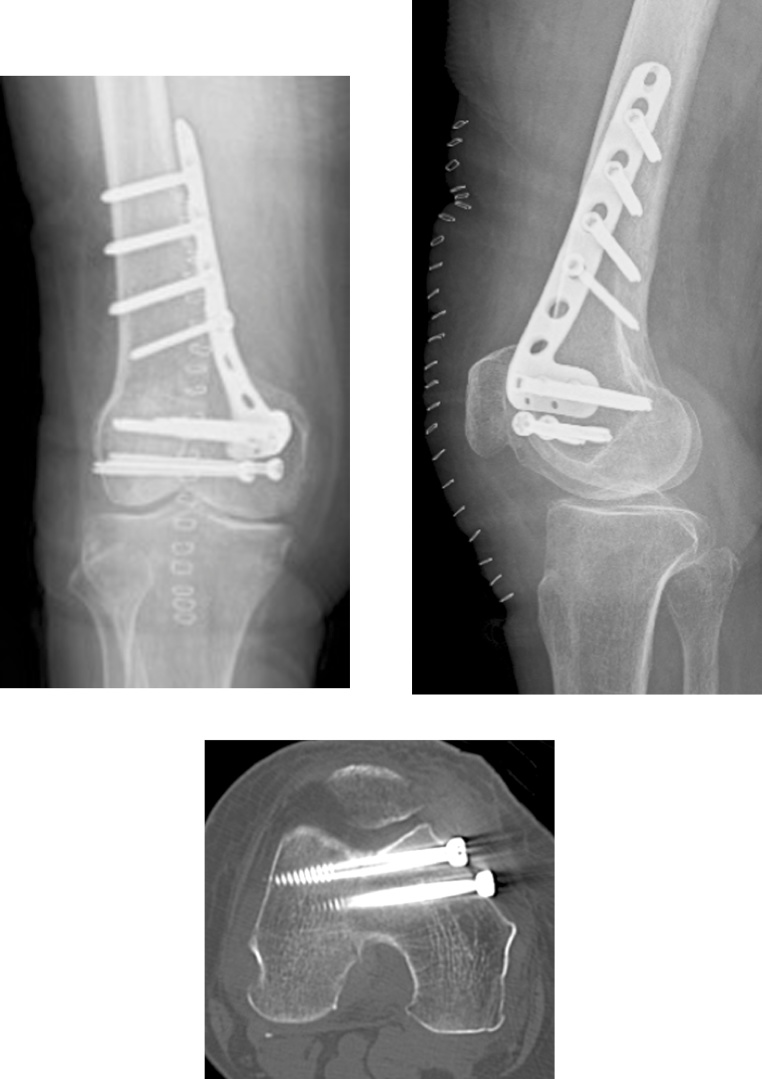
The post-operative plain radiography and computed tomography.
